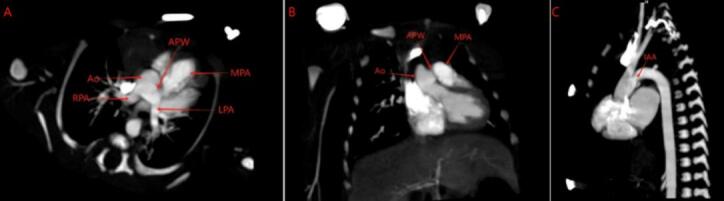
Computed tomography angiography (CTA) demonstrated type II aortopulmonary window (APW). The right pulmonary artery (RPA) arose from the ascending aorta (Ao). The left pulmonary artery (LPA) had normal origin from the main pulmonary artery (MPA). The aortic arch was interrupted distal to the left subclavian artery (IAA).
